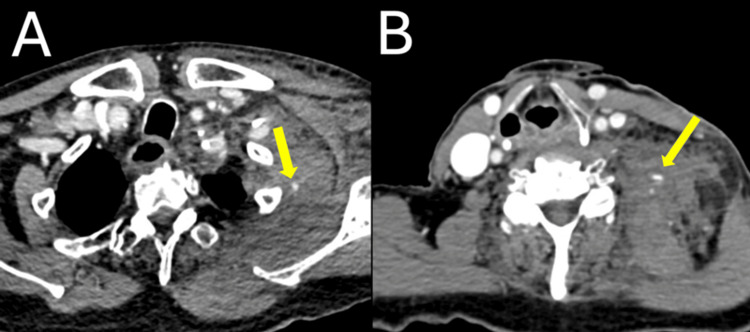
CT of the neck - soft tissue with contrast. This CT demonstrates a large left neck hematoma with extension inferiorly into the left chest wall and axilla with mass effect on adjacent structures. There are foci, indicated by the arrows, concerning for active hemorrhage within the chest wall (panel A) and the left neck (panel B).
